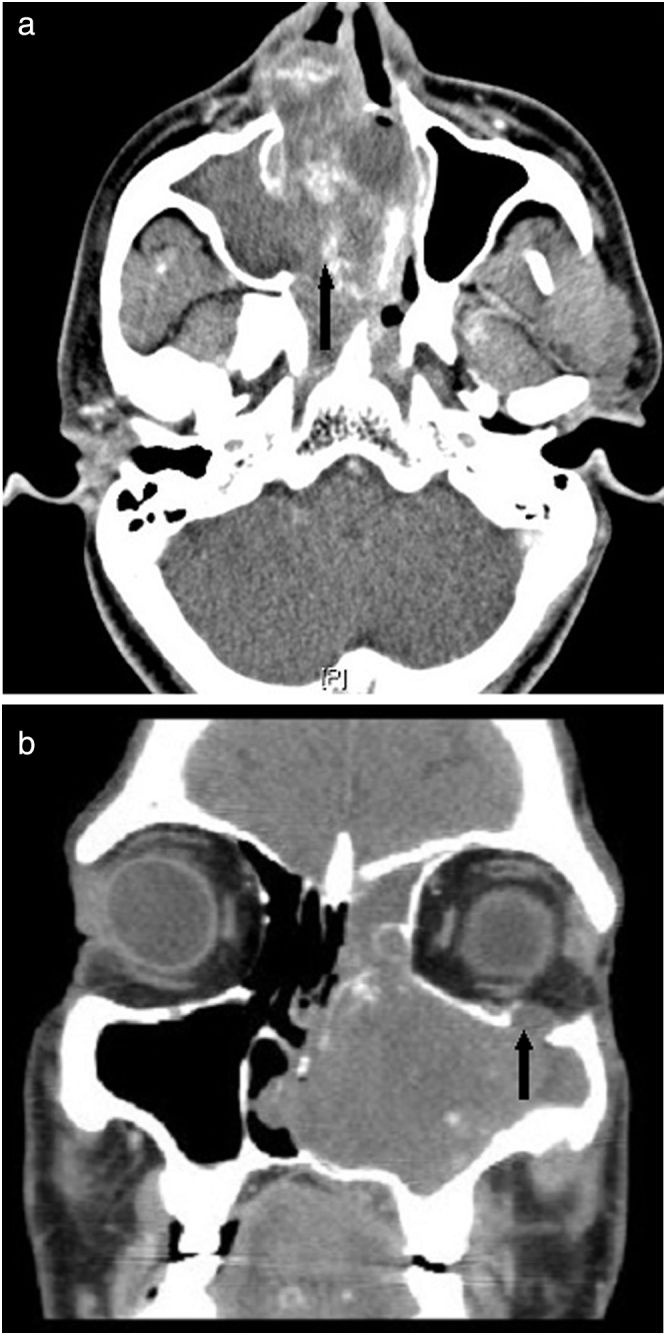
(a) Contrast-enhanced CT scan of the paranasal sinuses axial view showing heterogeneous, soft tissue opacification (black arrow) filling the right maxillary sinus and right nasal cavity pushing the septum to the left. (b) CT scan of the paranasal sinuses coronal view showing expansion of the left maxillary sinus cavity, bone remodelling, widening of the infundibulum and erosion of the orbital floor (black arrow).
